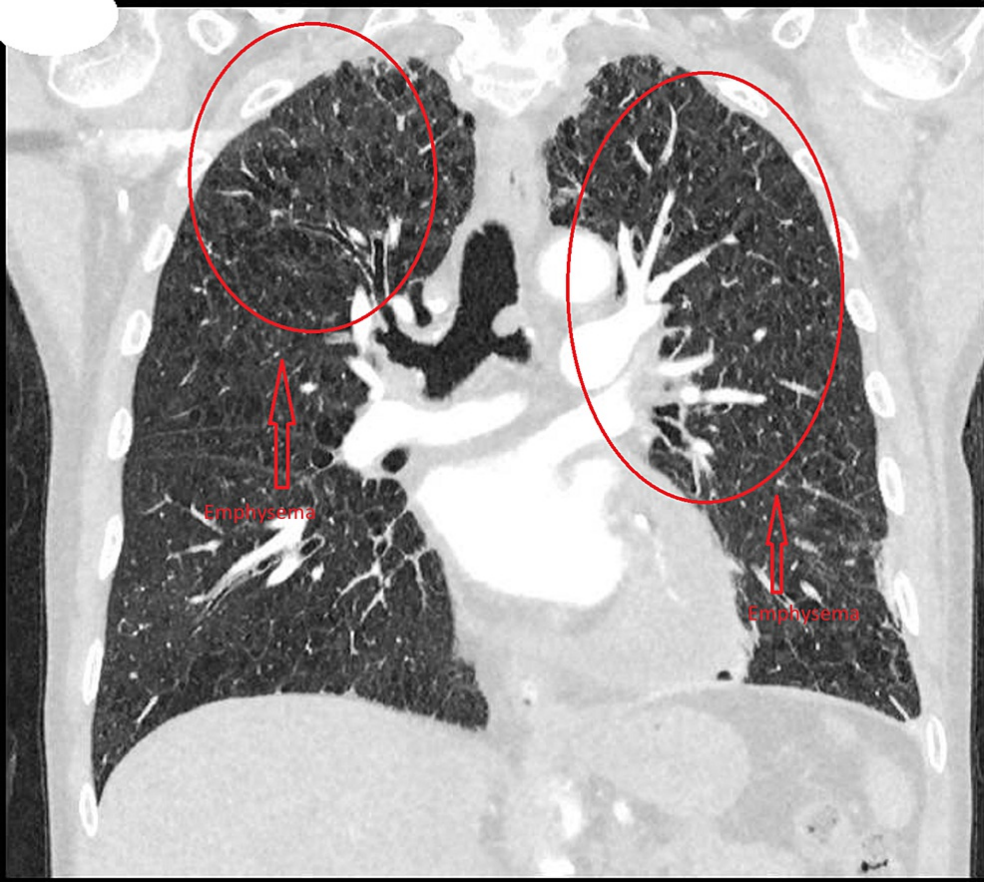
CT pulmonary angiogram CT pulmonary angiogram showing emphysema and no pulmonary embolus
